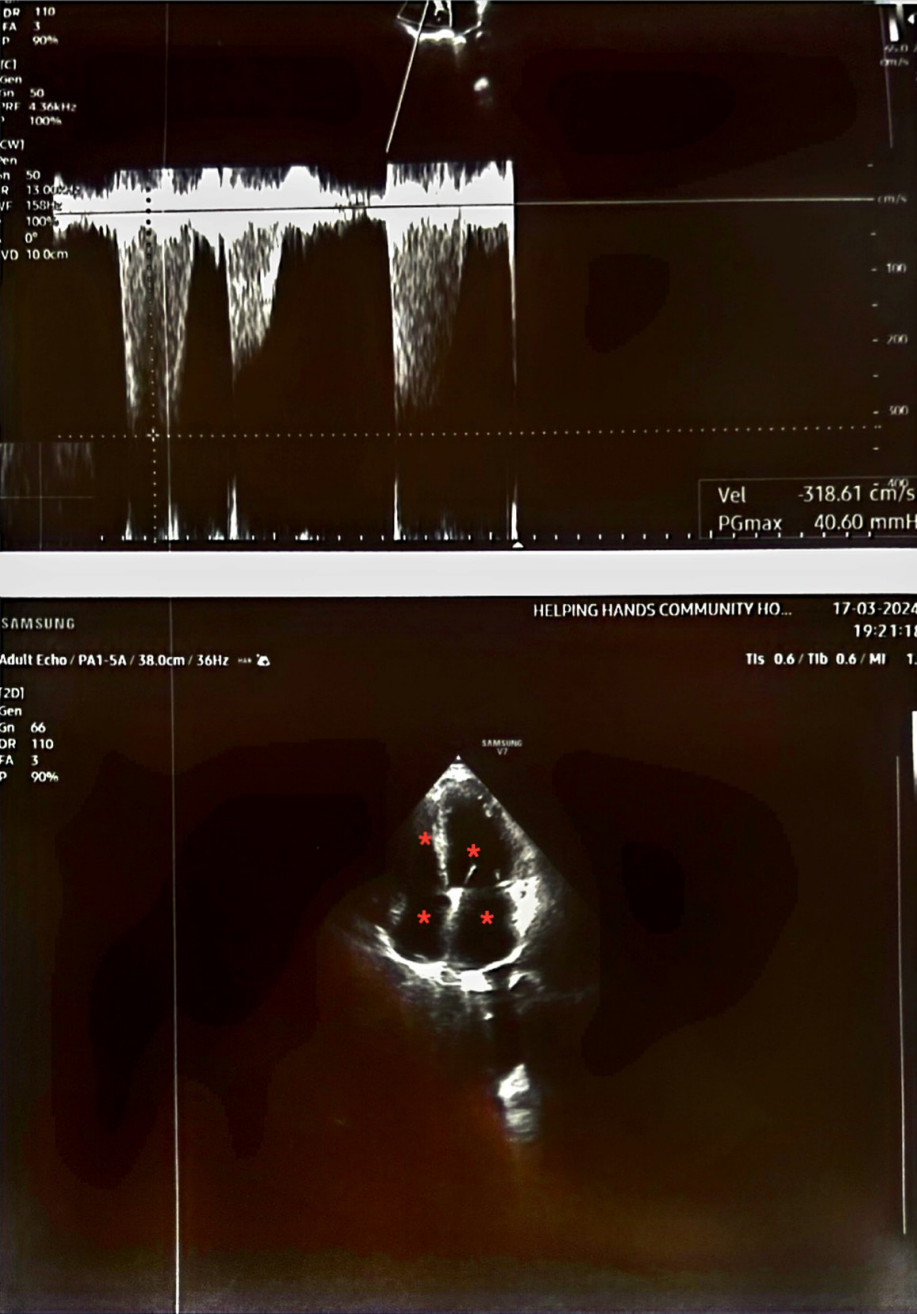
Echocardiography shows Dilated all chambers (marked by *), Global Left Ventricular wall hypokinesis, Moderate Mitral Regurgitation, Mild Aortic Regurgitation, Mild concentric Left Ventricular Hypertrophy, Moderate Tricuspid Regurgitation, Right Ventricular Systolic Pressure = 50 mmHg, Left Ventricular Diastolic Dysfunction, Moderate Left Ventricular Systolic Dysfunction, LVEF (Left Ventricular Ejection Fraction) = 30%–35%.
